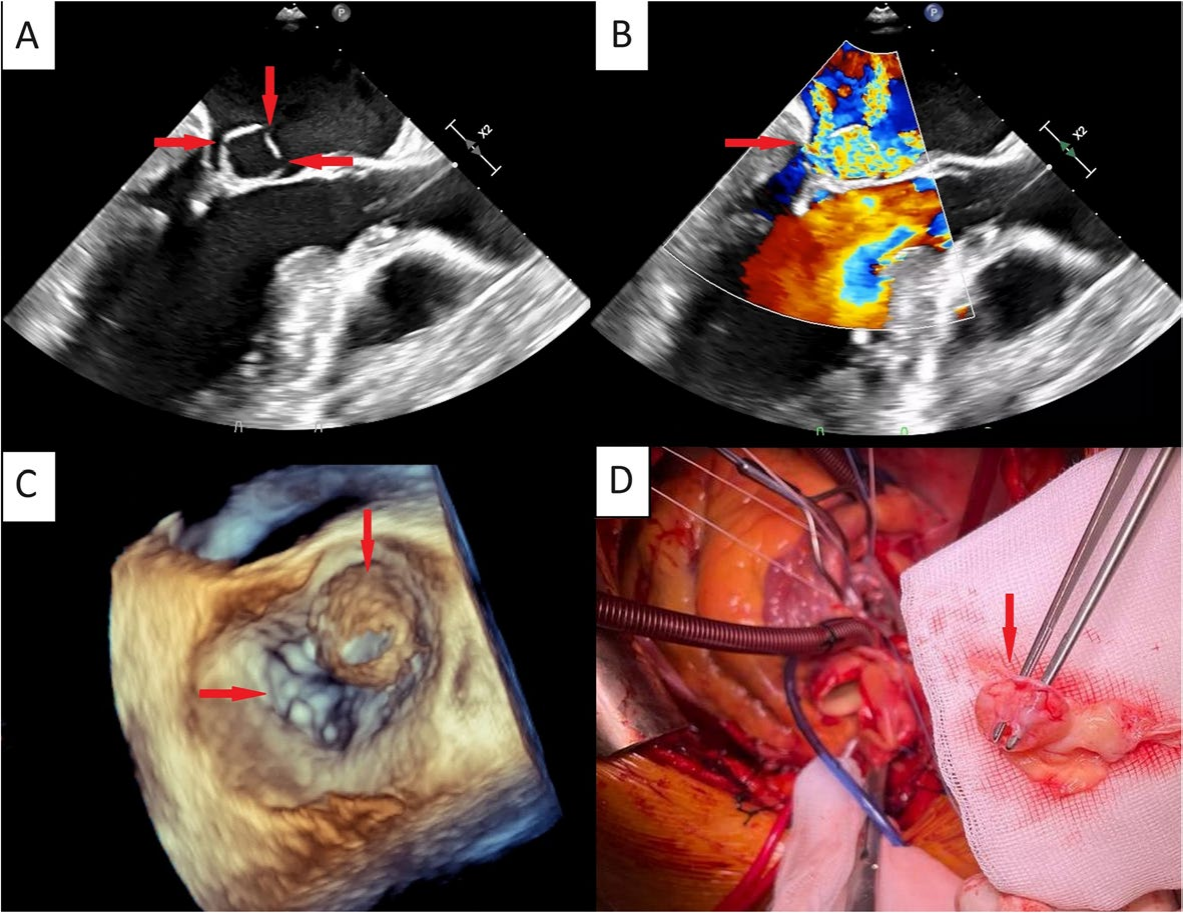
Transesophageal echocardiography revealed: A perforated abscess of the anterior mitral valve leaflet; B color turbulence due to wave of regurgitation from the aortic valve filling the perforated capsule. Three-dimensional echocardiographic imaging showed: C perforated capsule within the mitral valve; D intraoperative picture of the capsule
